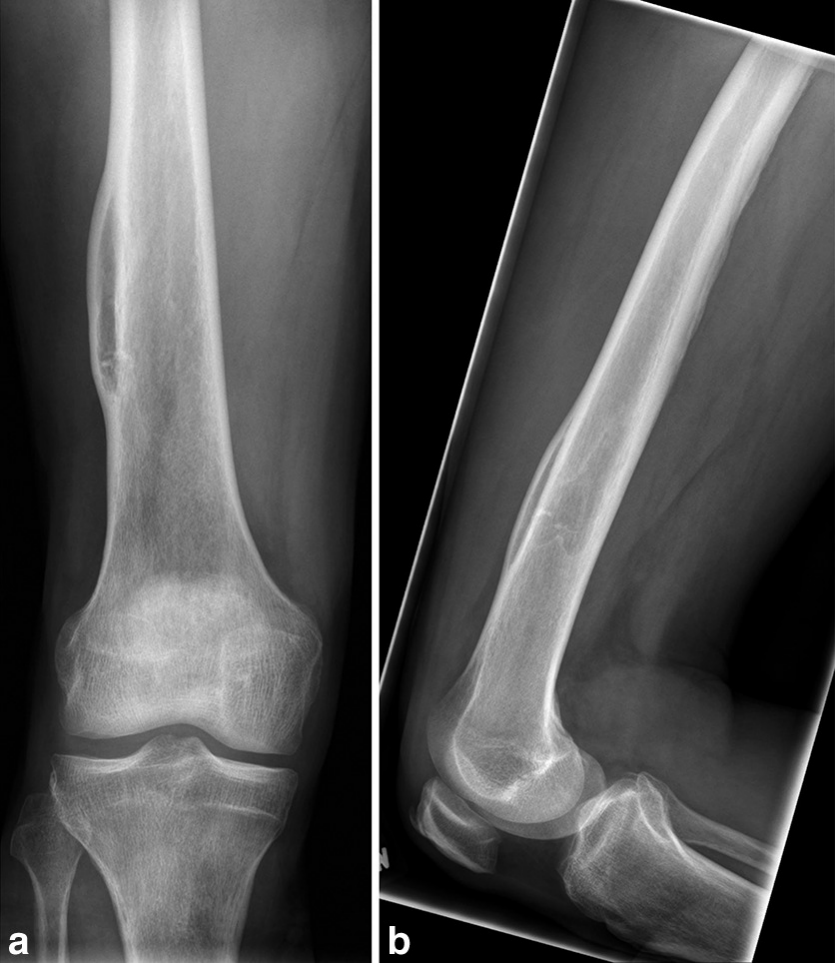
(a) Anterior–posterior and (b) lateral plain radiographs of the distal right femur.
